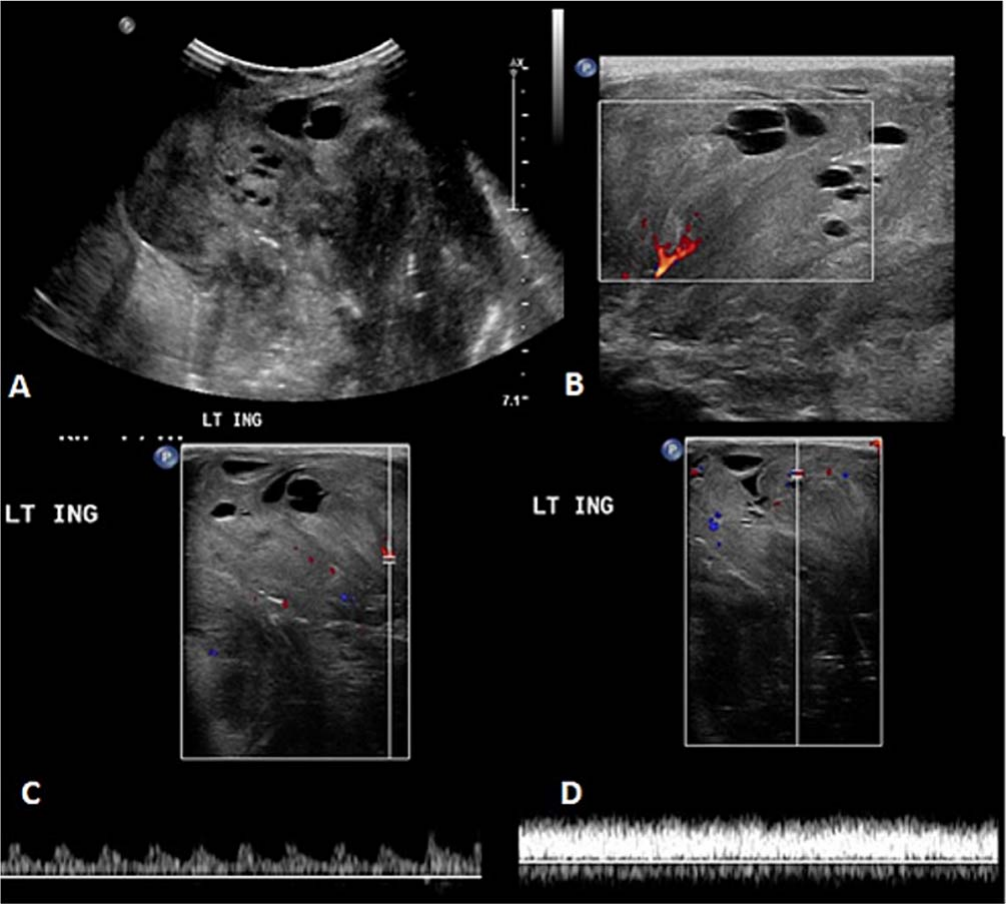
The follow-up ultrasound 3 weeks after MRI. (A) The gray scale image demonstrates a large heterogeneous predominantly isoechoic mass seen at the left inguinal region with multiple scattered areas of cystic changes and difficult-to-assess extent due to its size. (B–D) Color Doppler and spectral wave images show vascularity within the mass with detectable arterial and venous waveform.
